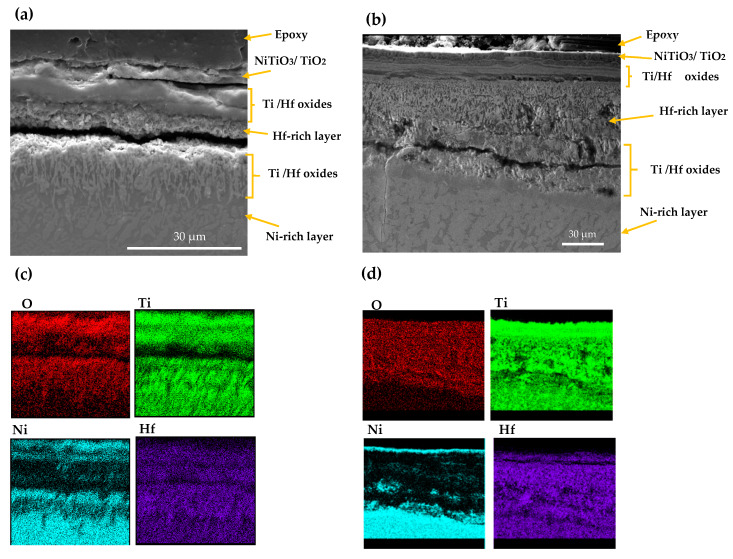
SEM image and X-ray mapping of the cross section of oxidation scale. (a,c) AM NiTiHf at 800 °C; (b,d) AM NiTiHf at 900 °C.
